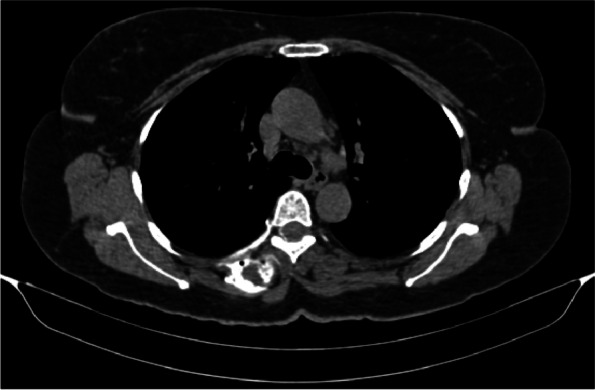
Axial CT scan demonstrating epidural spread of LA-dye mixture at T5 level
